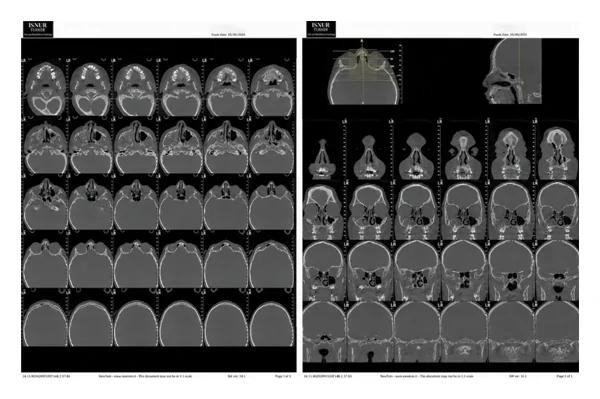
CBCT images of the paranasal sinuses in axial and coronal planes.
